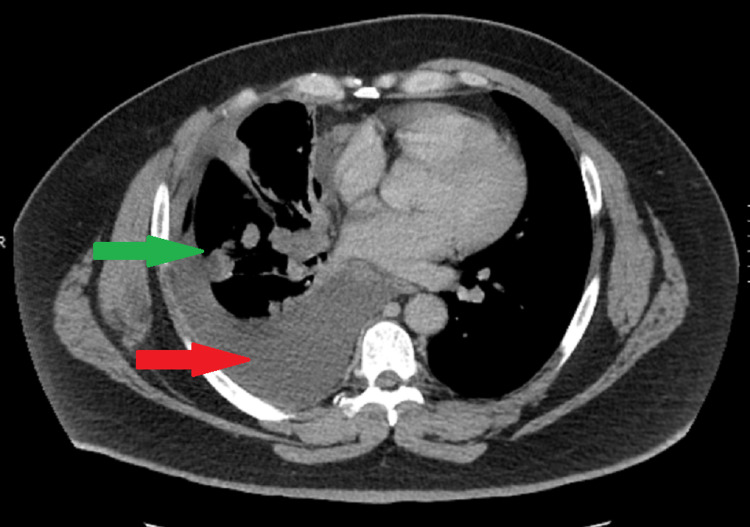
CT scan showing right-sided lung mass (green arrow) and pleural effusion (red arrow).
